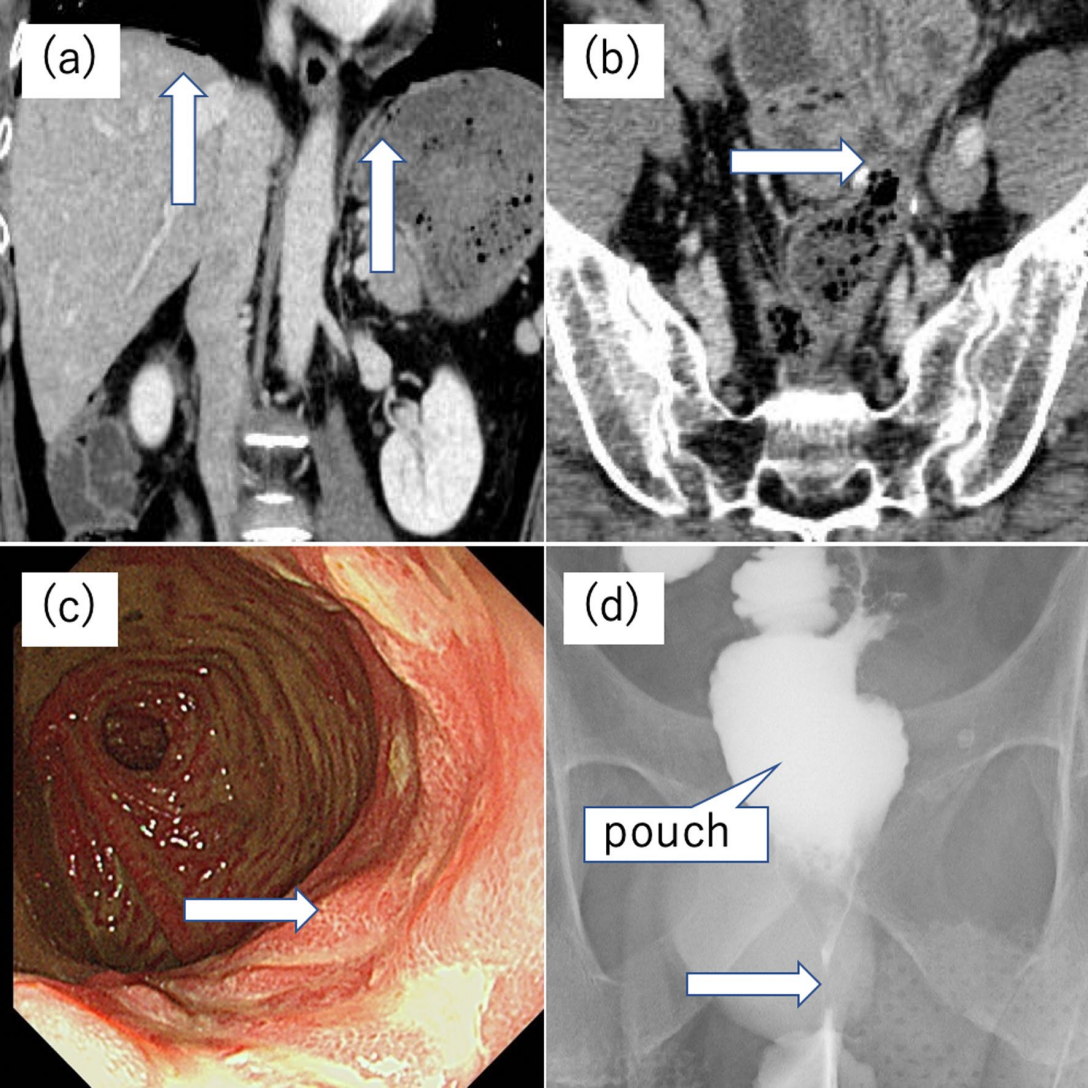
Findings upon the second perforation. a, b Contrast-enhanced computed tomography. Contrast-enhanced CT revealed free air (arrow: a) and fluid collection around the blind end of the J-pouch (arrow: b). c Endoscopic observation and gastrointestinal gastrography findings after surgery. Endoscopy revealed multiple ulcers in the ileal J-pouch, and gastrointestinal gastrography revealed an anastomotic stricture. d Gastrointestinal gastrographic findings after surgery. Gastrointestinal gastrography revealed an anastomotic stricture again
